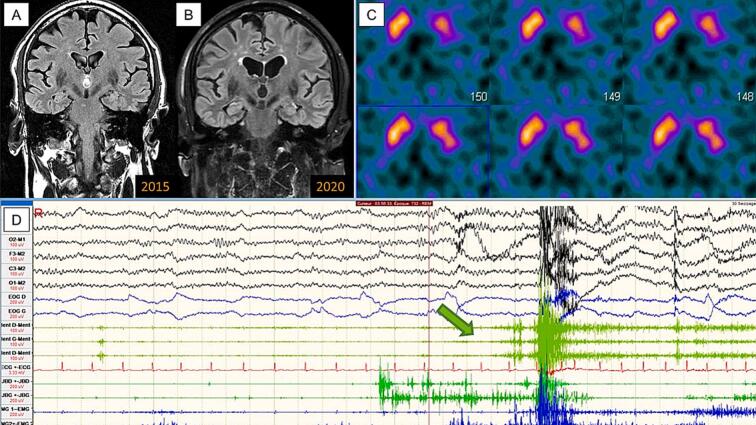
selected paraclinical features.*Intended for color reproduction on the Web.* Legend: A-B = brain MRI, baseline and 5-years follow-up matched T2-FLAIR coronal slices showing progressive cortical and subcortical atrophy; C = predominant left-sided dopaminergic denervation on FP-CIT acquisitions (DaT-Scan); D = polysomnography, loss of muscular tone in REM-sleep (green arrow). (For interpretation of the references to color in this figure legend, the reader is referred to the web version of this article.)
